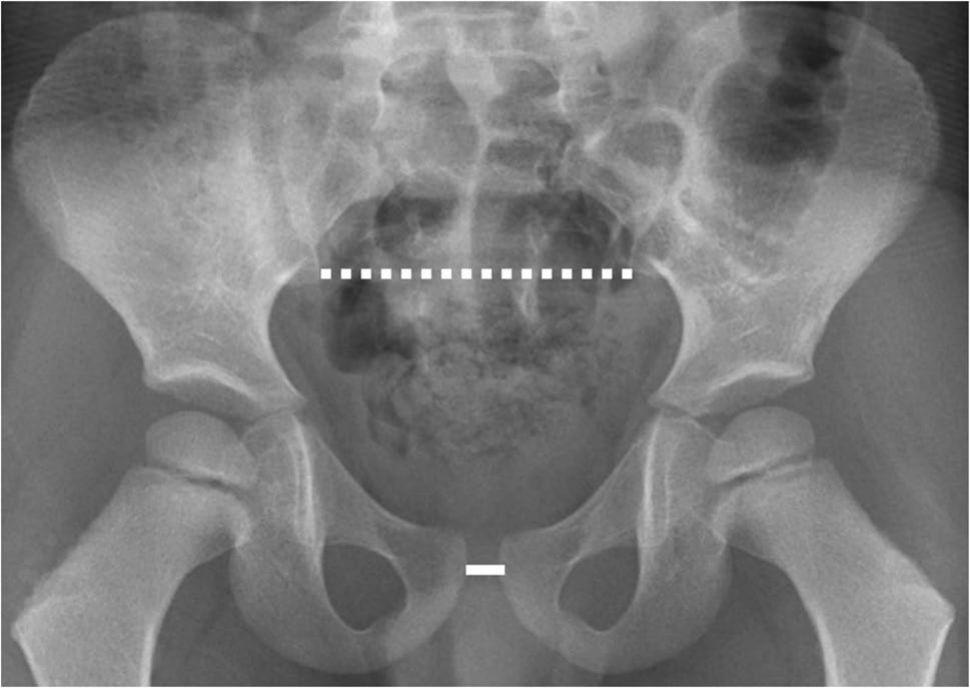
Pelvic ratio measurement example on a clinical radiograph. Normal anterior-posterior pelvic radiograph of a 4-year-old boy with knee pain. Dashed line indicates the distance between the posterior inferior iliac spines (the sacral width at a prescribed, recognizable location) and solid line indicates the inter-pubic distance. The pelvic ratio is the inter-pubic distance divided by the sacral width
